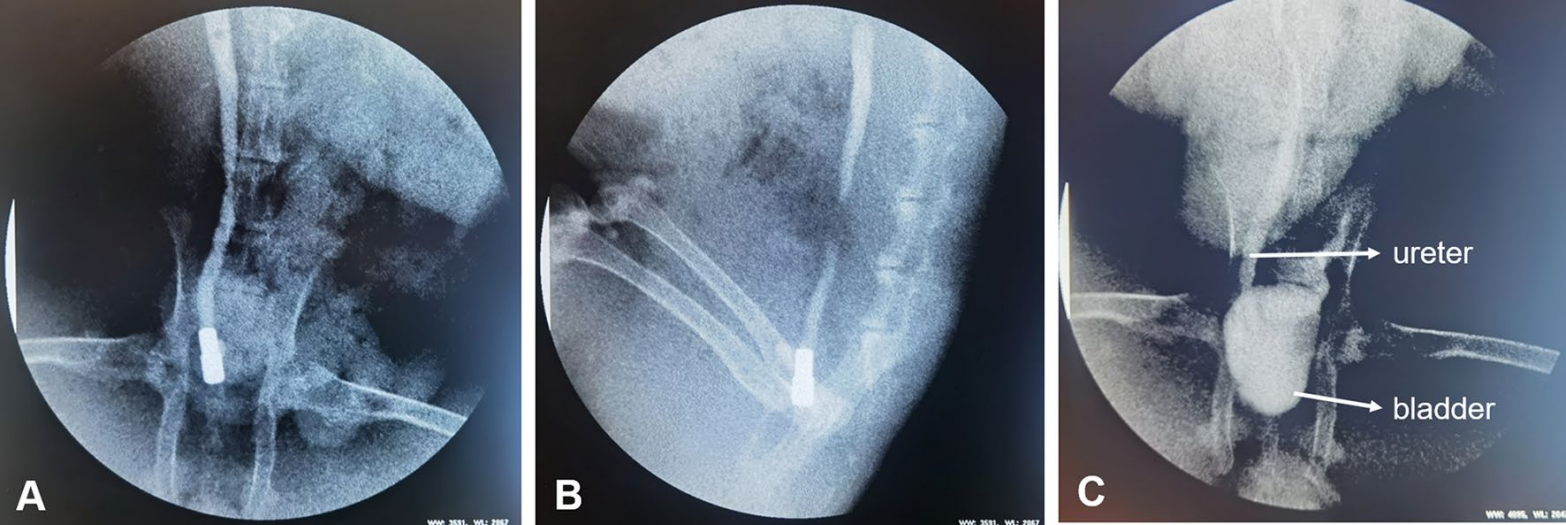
Confirmation of the position of the two magnets by ureterography. (A) Magnet location is shown on an abdominal radiograph; (B) Magnet location is shown on a lateral abdominal radiograph; (C) Right ureterography showing anastomotic patency.
